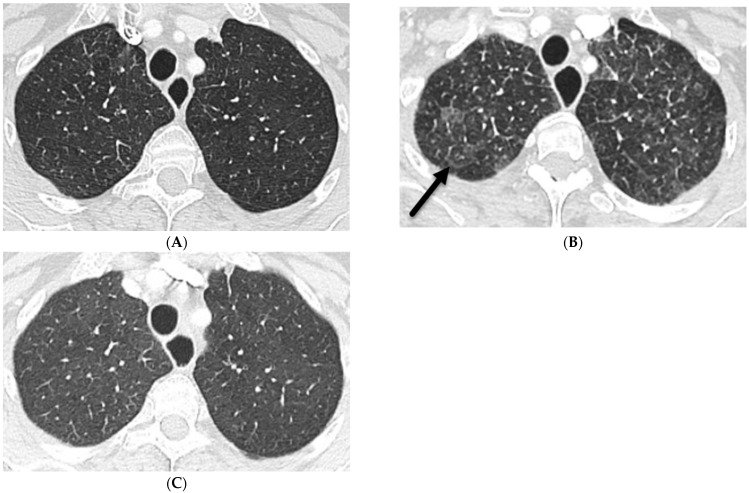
A 68-year-old female with a history of peritoneal mesothelioma. An axial chest CT image at baseline (A) shows clear lungs. An axial chest CT image 2 weeks after starting immunotherapy (B) demonstrates GGO, perilymphatic nodules, interlobular septal thickening (solid black arrow), and mediastinal and hilar lymphadenopathy (not shown) mimicking sarcoidosis. An axial chest CT image 2 months later after drug discontinuation corticosteroid treatment (C) shows near-complete resolution of pulmonary abnormalities.
